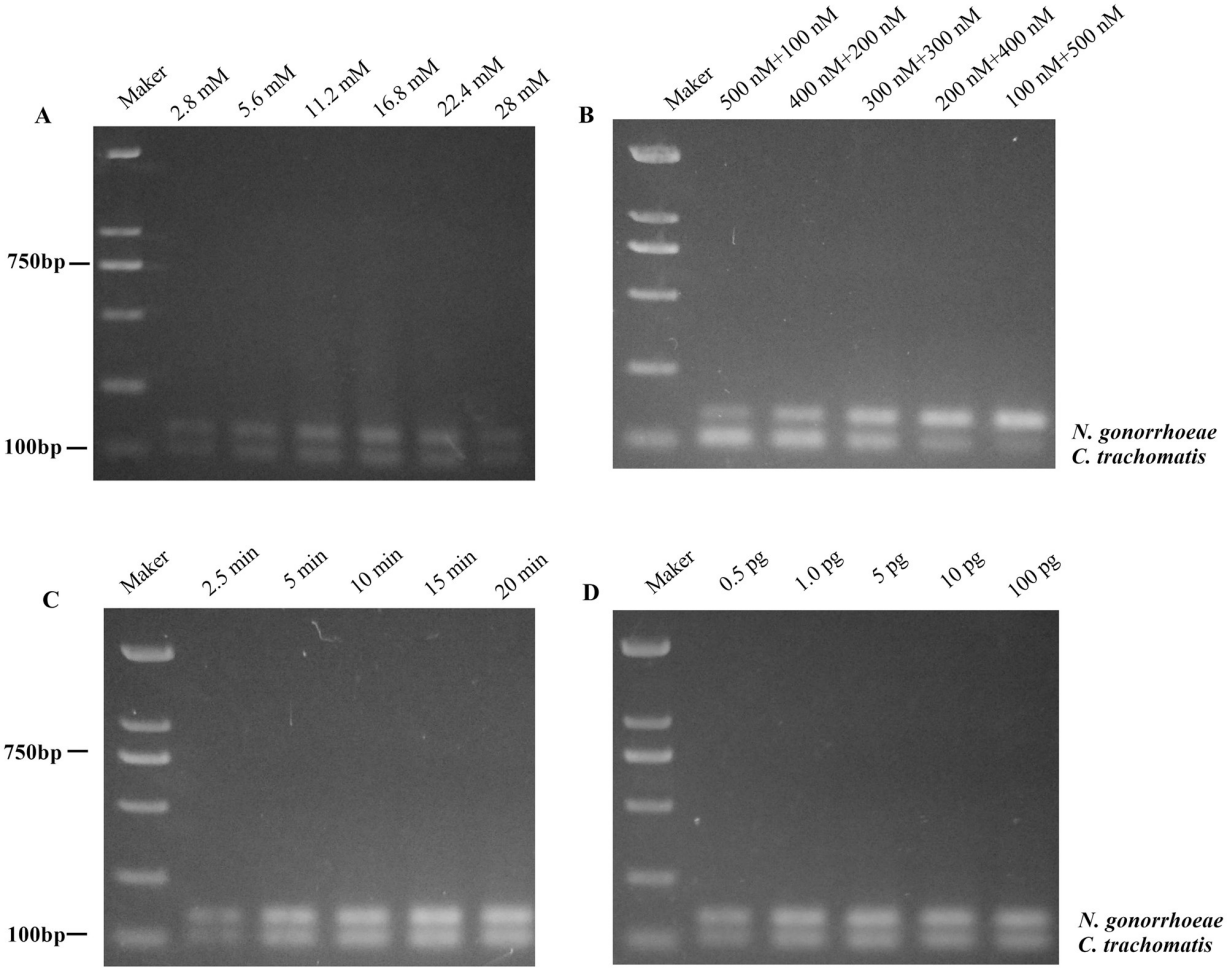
Optimization of multiple RPA reaction system. A: Comparison of amplification efficiency of multiplex RPA at different magnesium ion concentrations (38°C, 20 min, CT 200 nM + NG 200 nM); B: Comparison of amplification efficiency of multiplex RPA at different combinations of primer pair concentrations (38°C, 20 min, 16.8 nM Mg^2+^); C: Comparison of amplification yields of multiplex RPA at different amplification times (38°C, 16.8 nM Mg^2+^, CT 300 nM + NG 300 nM); D: Comparison of amplification efficiency of multiplex RPA at different template concentration conditions (38°C, 10 min, 16.8 nM Mg^2+^, CT 300 nM + NG 300 nM).
